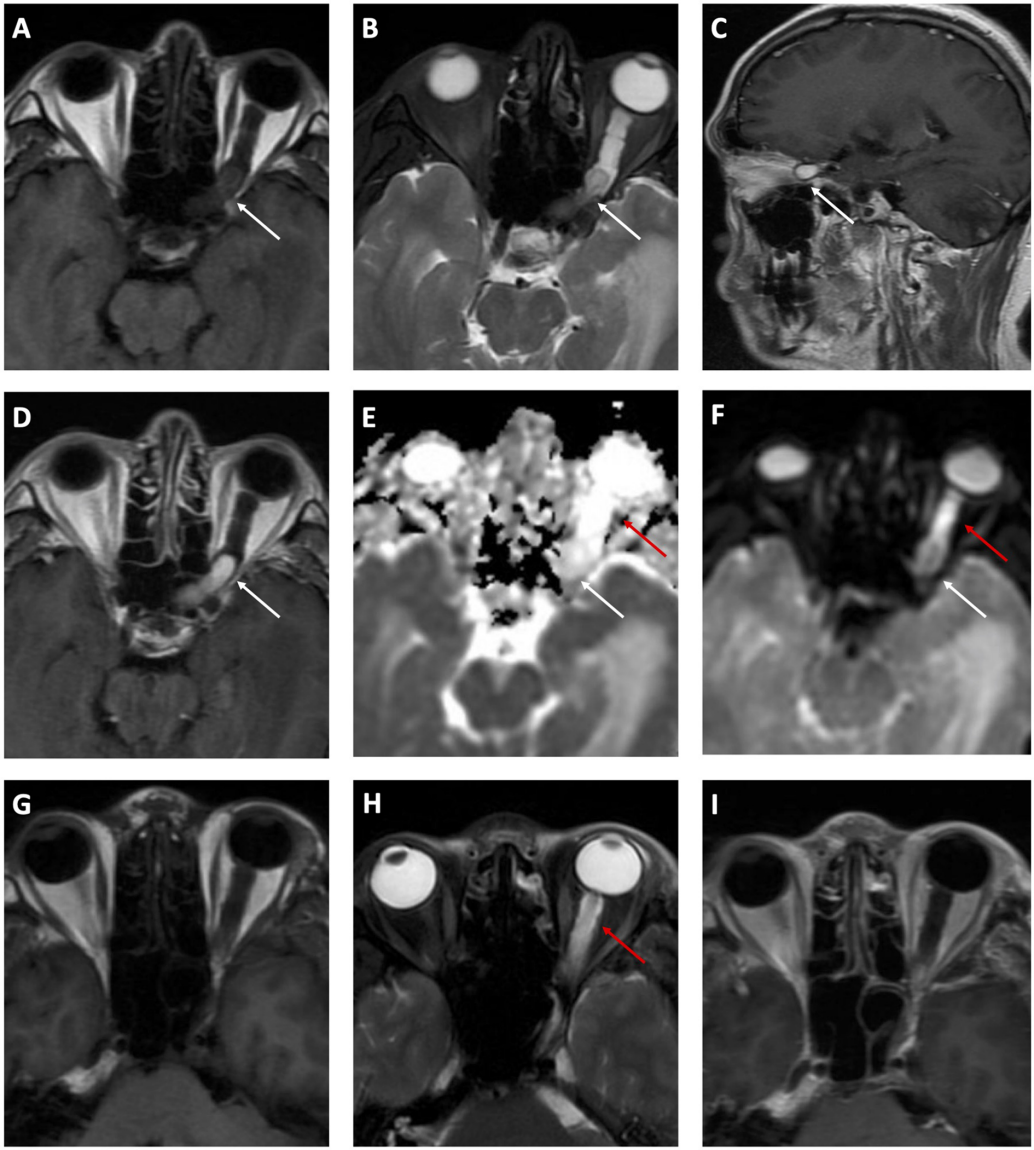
Preoperative (A–F) and postoperative (G–I) MRI. A hypointense mass (white arrows) from the left orbit apex to the anterior cranial fossa is shown on T1-weighted axial (A) MRI scan. It is hyperintense on T2-weighted axial (B) and sagittal (C) MRI scans, with a significant enhancement on contrast images (D). Obvious hyperintensity is identified on the ADC map (E) but not on DWI (F). The mass is absent on both T1WI (G) and T2WI (H) while edema (red arrows) recedes incompletely. The contrast scan (I) shows no enhancement indicating the mass was excised completely.
